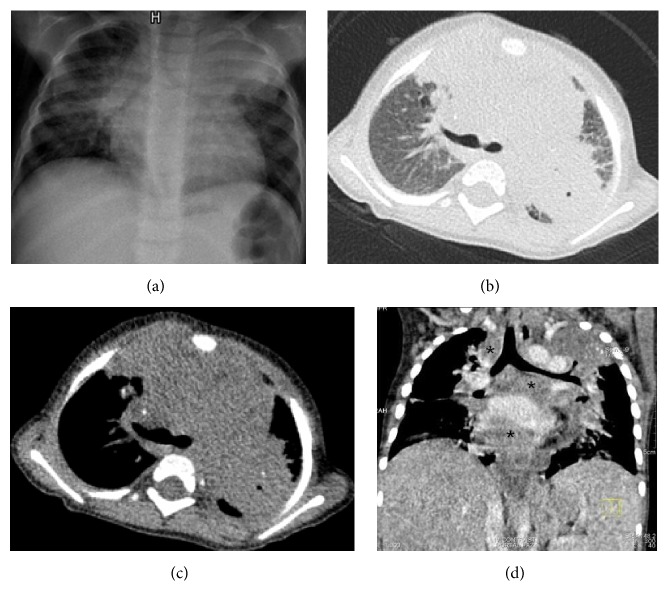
(a) Chest X-ray: Bilateral extensive infiltrations especially prominent in left upper lobe. (b) and (c) Thorax CT (parenchymal and mediastinal window, resp.): Right upper lobe anterior segment, left upper lobe nearly total consolidations. In particular the consolidation in left upper lobe and right paratracheal, hilar region includes calcifications. (d) Thorax CT (two months later, coronal section): Extensive visceral and parietal lympadenomegalies in mediastinum that reach 3 cm in diameter, some showing central necrosis especially the ones in pericardial neighborhood (*∗*).
